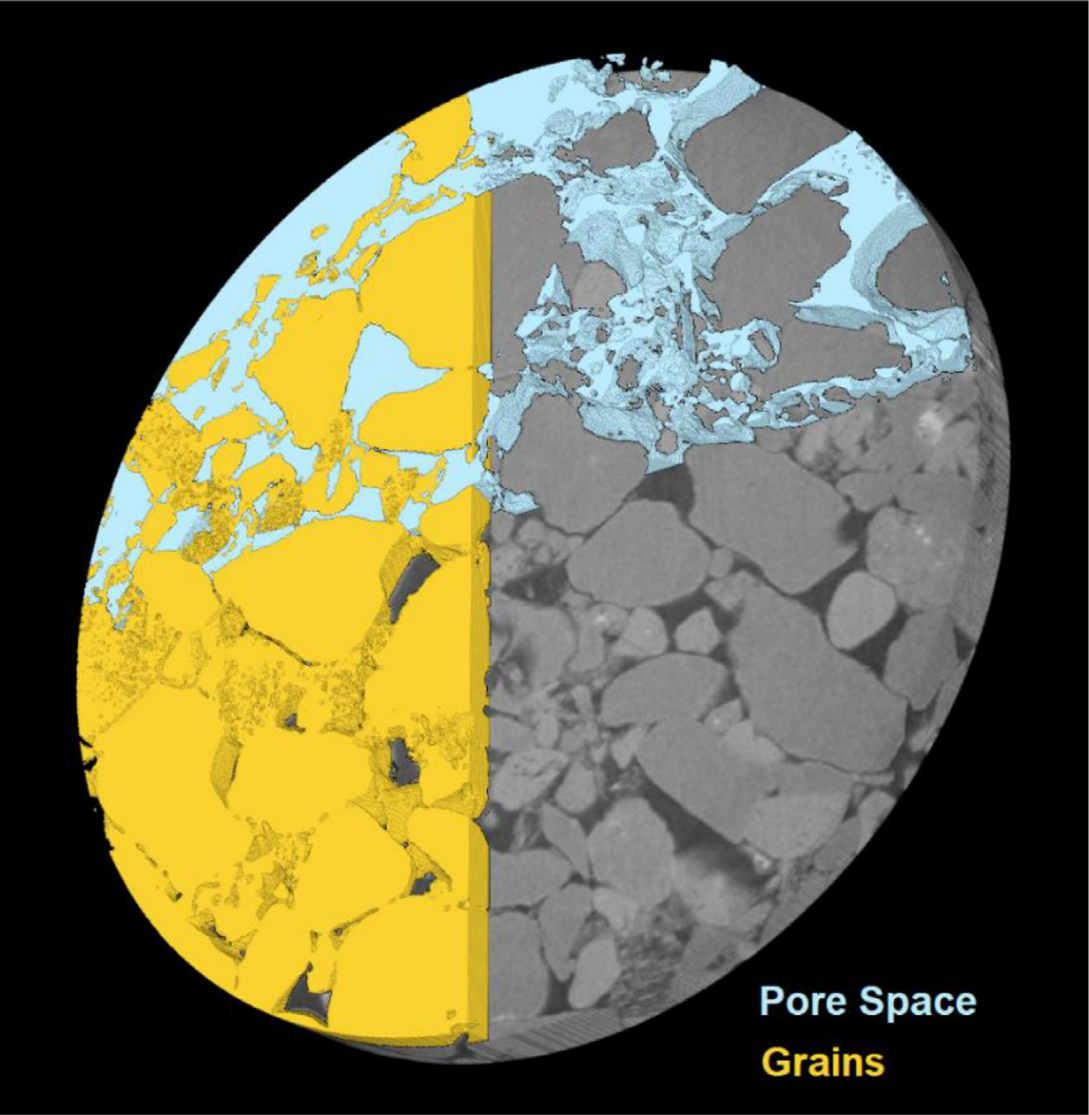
Segmentation: example of pore space and matrix grain isolation in a 2.1 mm diameter digital representation of scan 2-Mt.Simon_6986ft_10x. Pore space is shown in blue, and matrix grains in yellow, with original computed tomography data seen in the background in grayscale.
